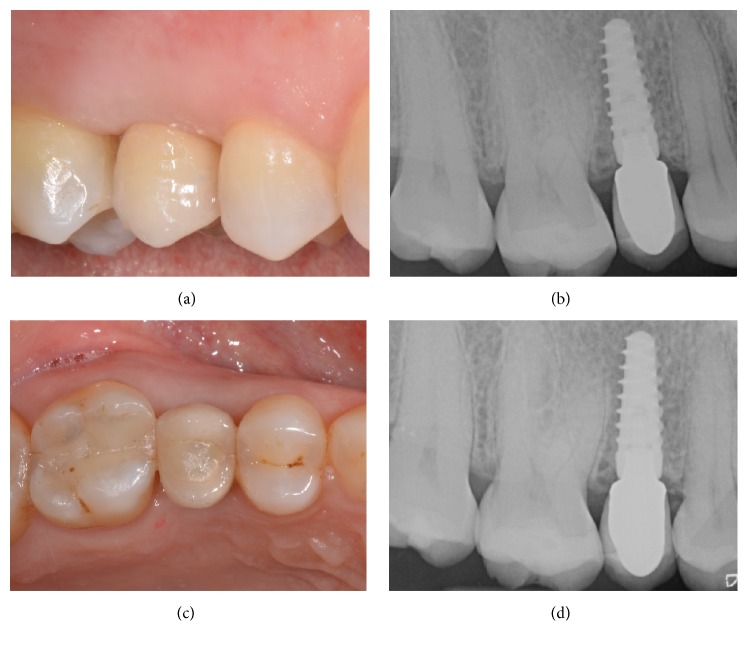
Delayed implant placement of a maxillary second premolar (#15). Final metal-ceramic crown at delivery, lateral view (a); periapical radiograph of the implant at the delivery of the final crown (b); final metal-ceramic crown at delivery, occlusal view (c); periapical radiograph 2 years after implant insertion (d).
